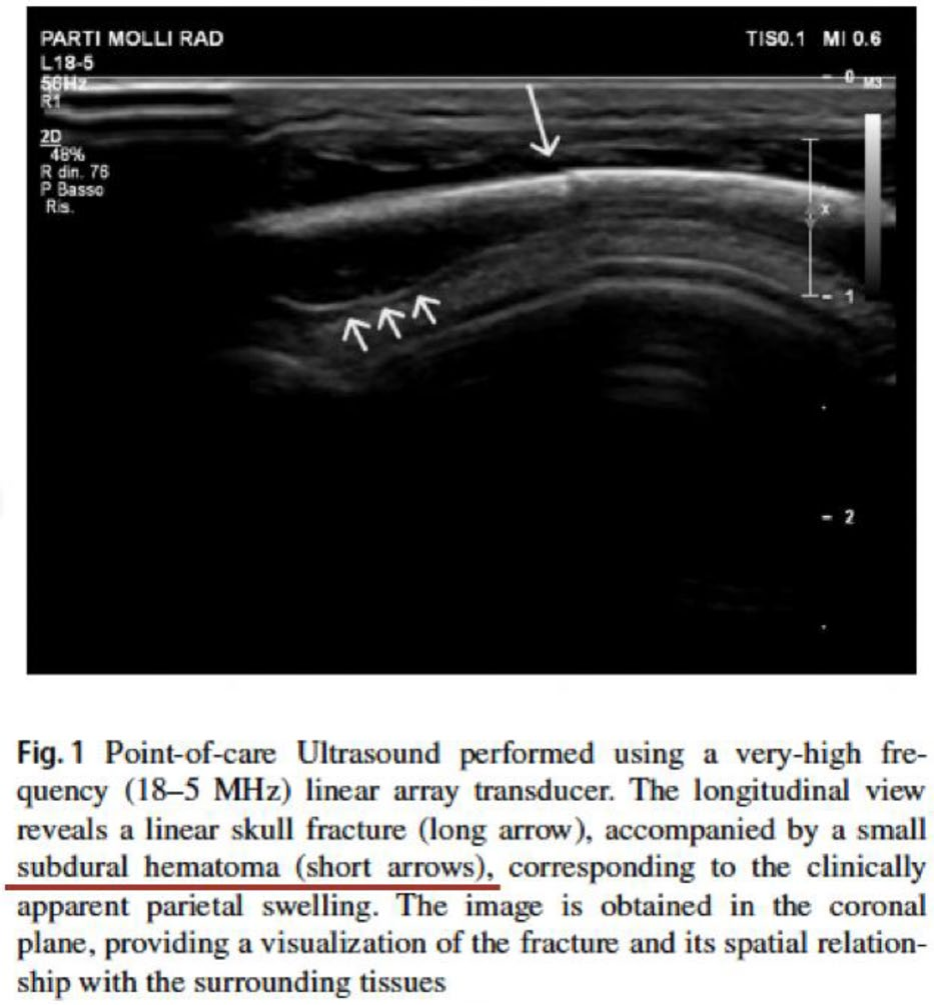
# Re: Identifying skull fractures after head trauma in infants with ultrasonography: is that possible?
